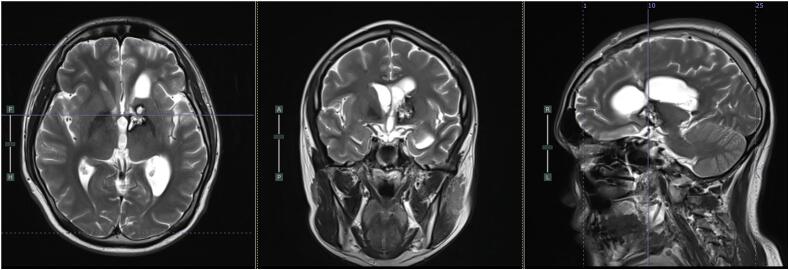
T2 weighted MRI showed iso to hyperintense mass in the basal ganglia sizing 2.2 × 1.2 × 2.2 cm. The mass appears smaller compared to the previous imaging.
